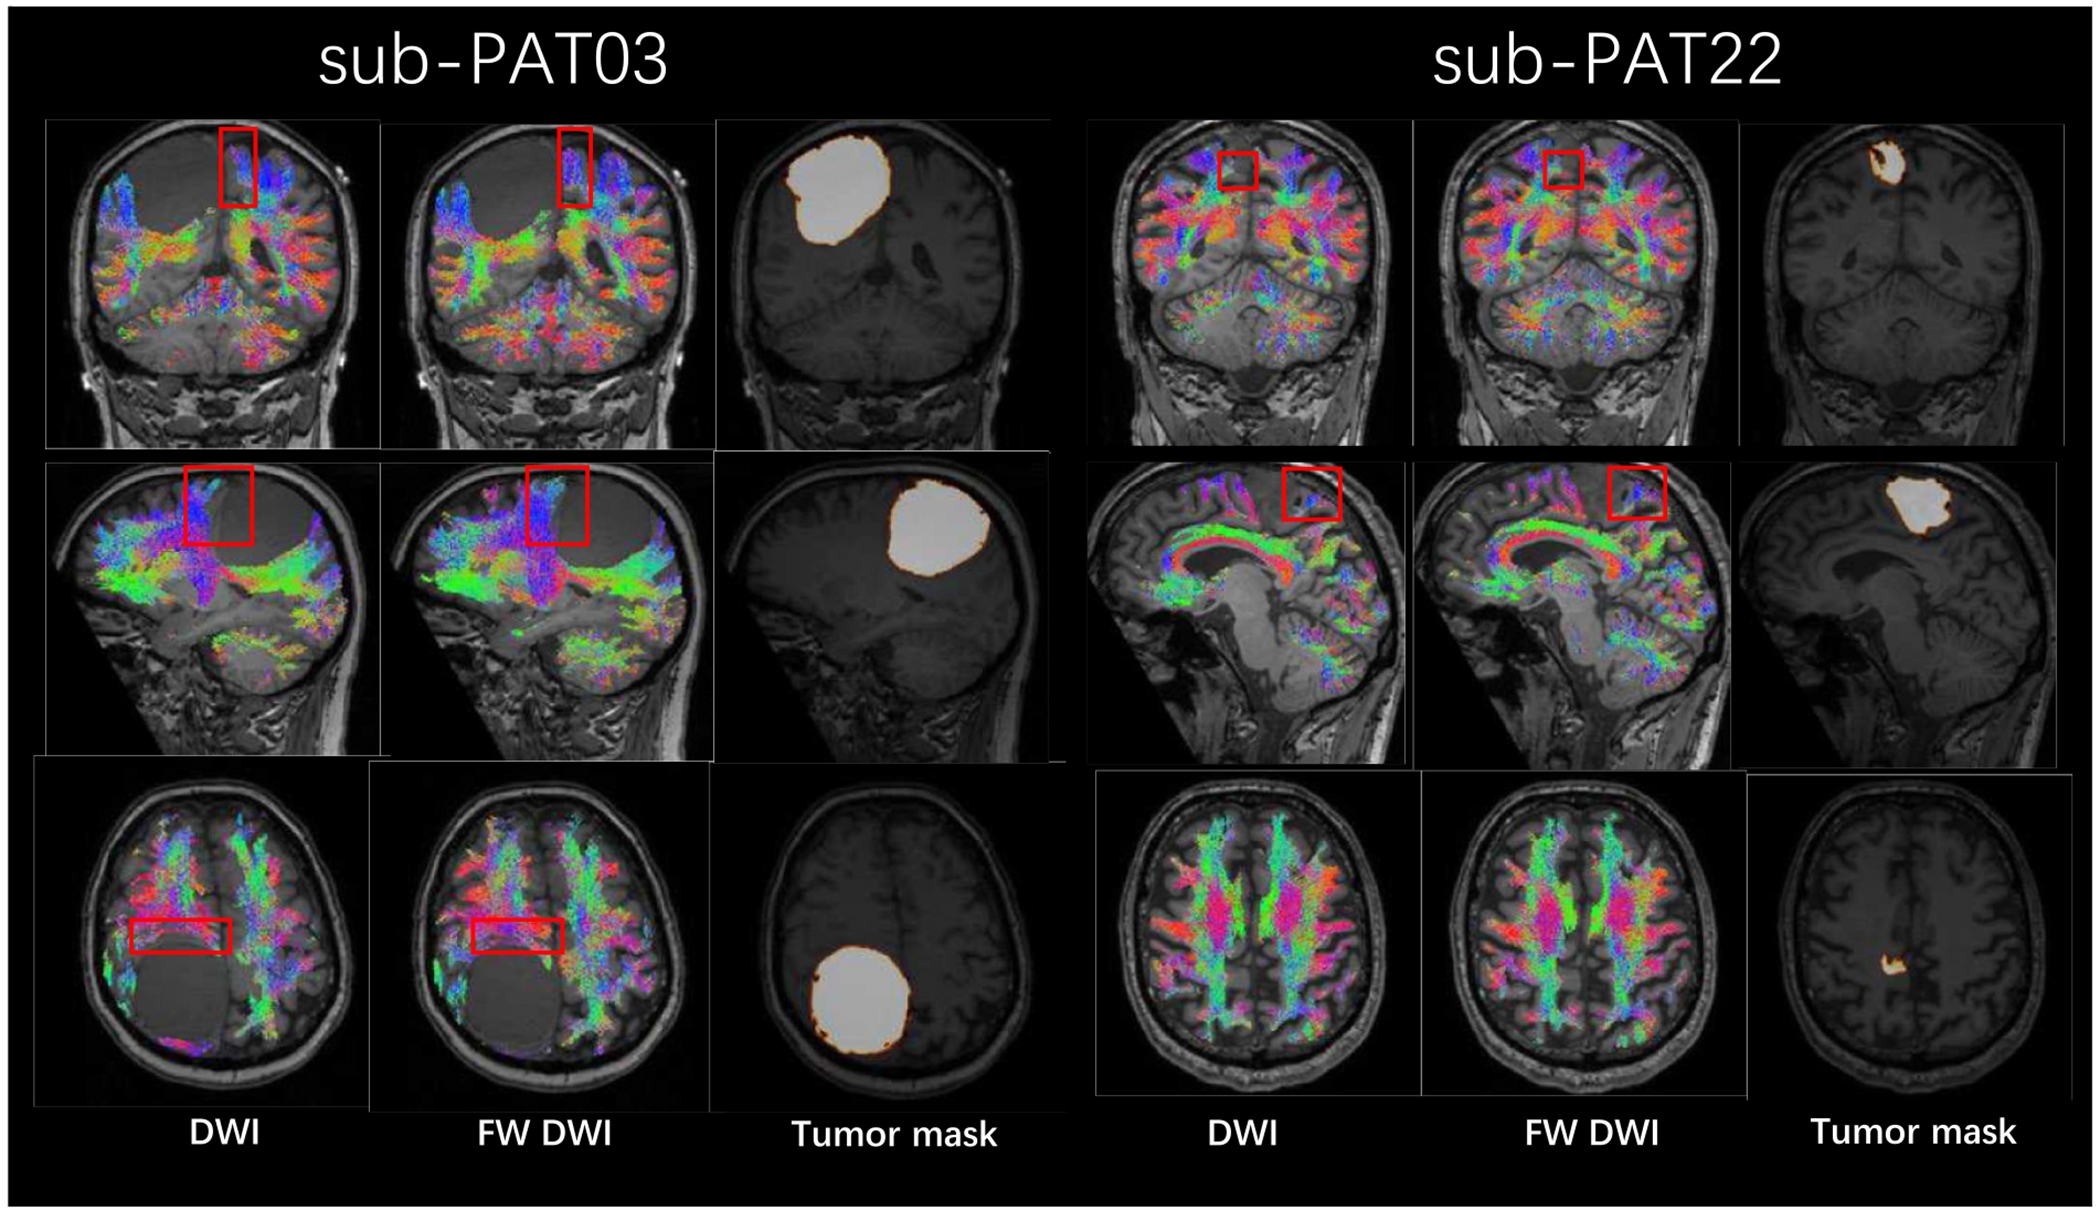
Comparison of tractography results before and after free water elimination for two representative patients: Sub-03, a 60-year-old female with meningioma type I in the parietal brain (tumor size: 78.44 *cm*^3^), and Sub-22, a 53-year-old male with oligodendroglioma type II in the parietal brain (tumor size: 15.16 *cm*^3^). The images display the original DWI (left), FW-corrected DWI (middle), and tumor mask (right). Red boxes highlight regions with notable differences, predominantly in areas affected by tumors, as indicated by the tumor mask. (For interpretation of the references to colour in this figure legend, the reader is referred to the web version of this article.)
